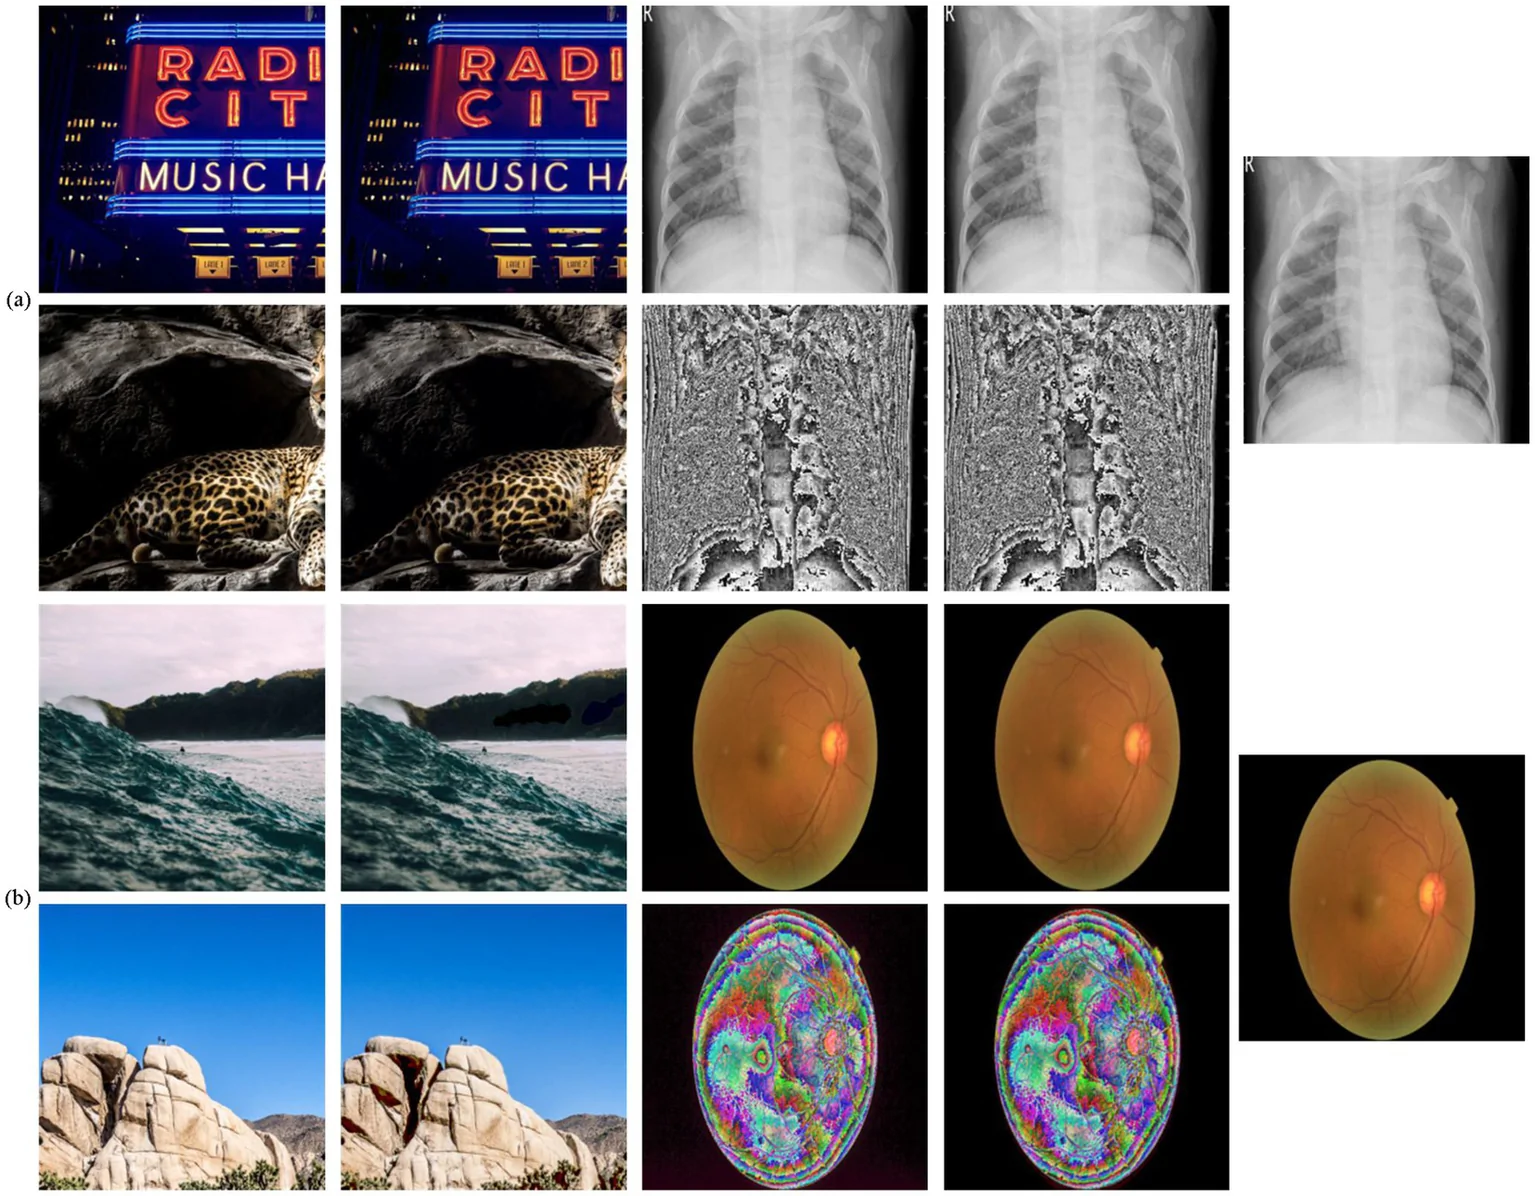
Visualization of hiding and recovery results on different datasets: (a) Chest X-ray, (b) Fundus imaging. Columns 1–5 correspond to the container, cover, embedded secret (or its secretcode), recovered secret (secretcode) image, and the losslessly recovered image, respectively.
